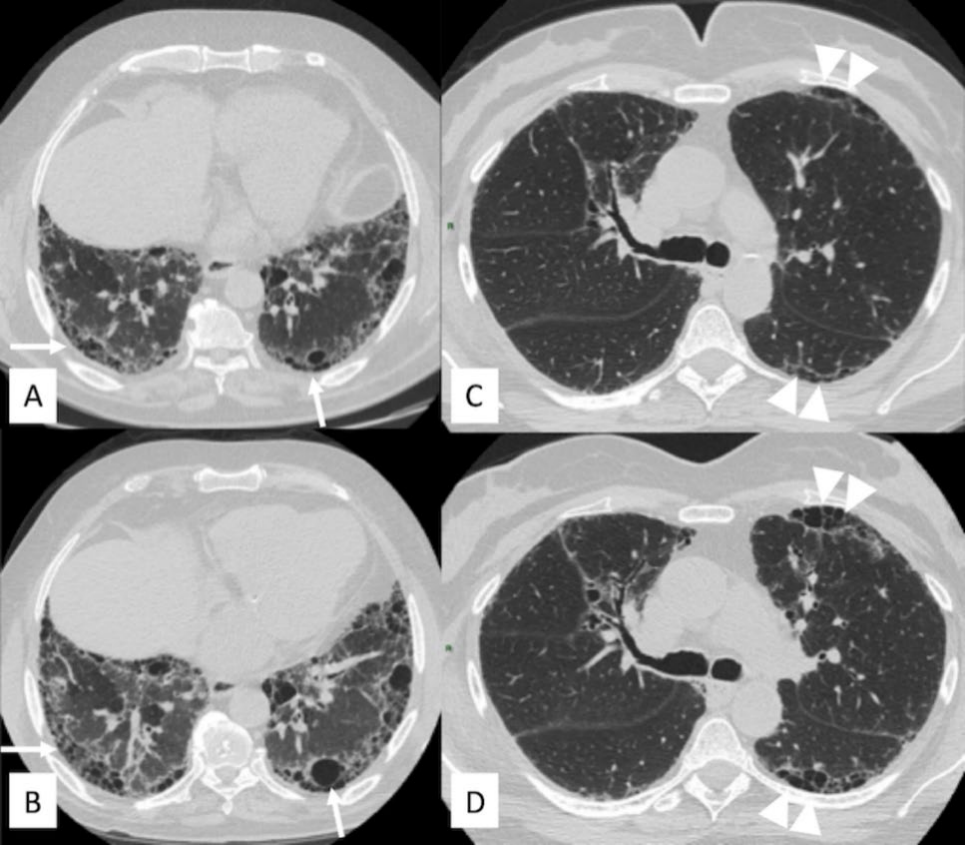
Progression of honeycombing in two different patients. Patient with fibrotic chronic hypersensitivity pneumonitis (CHP) with areas of honeycombing (white arrows) in the lower lobes at A baseline and B after 16 months of follow-up, where an increase in extent and severity of honeycombing are depicted on the periphery of both lower lobes. Patient with fibrotic connective tissue disease (CTD) with honeycomb spaces (white arrowheads) in the anterolateral region of the left upper lobe and in the posterosuperior region of the left lower lobe at C baseline and D after 12 months of follow-up: honeycomb is more represented in the follow-up high-resolution computed tomography examination
